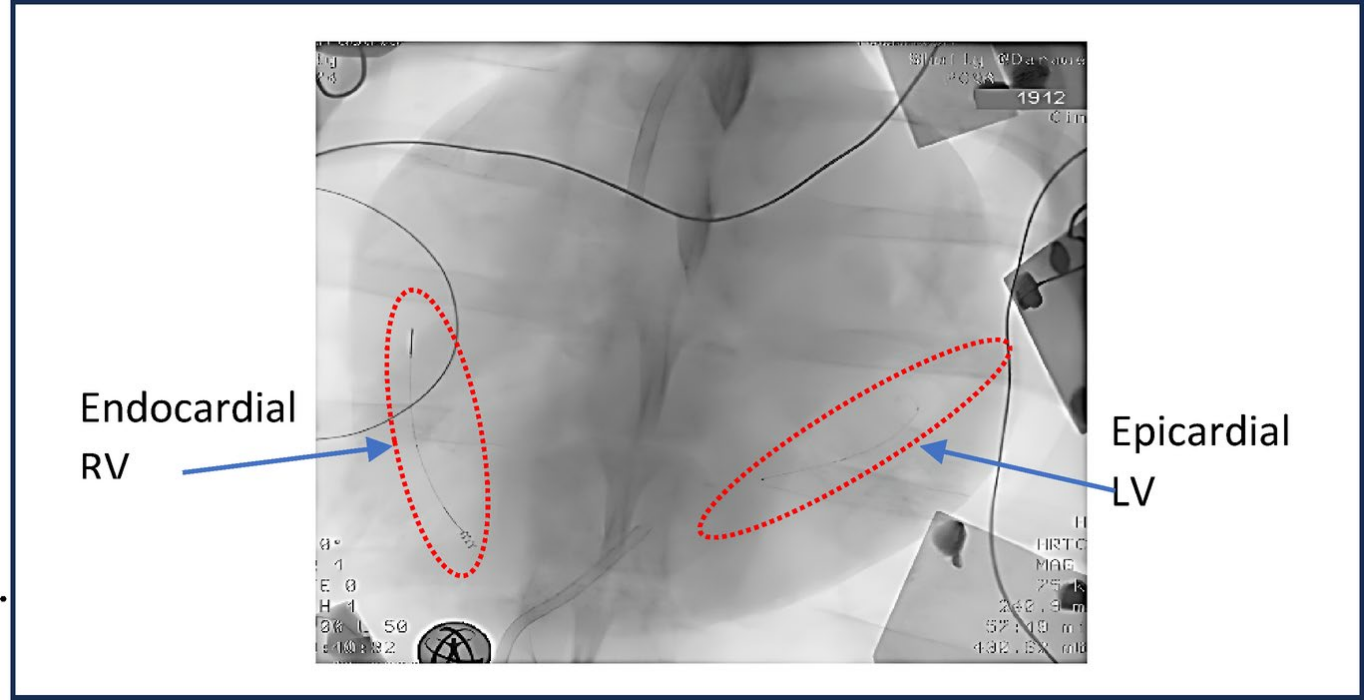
X-ray imaging after implanting endocardial RV and epicardial LV rectifier electrodes.
